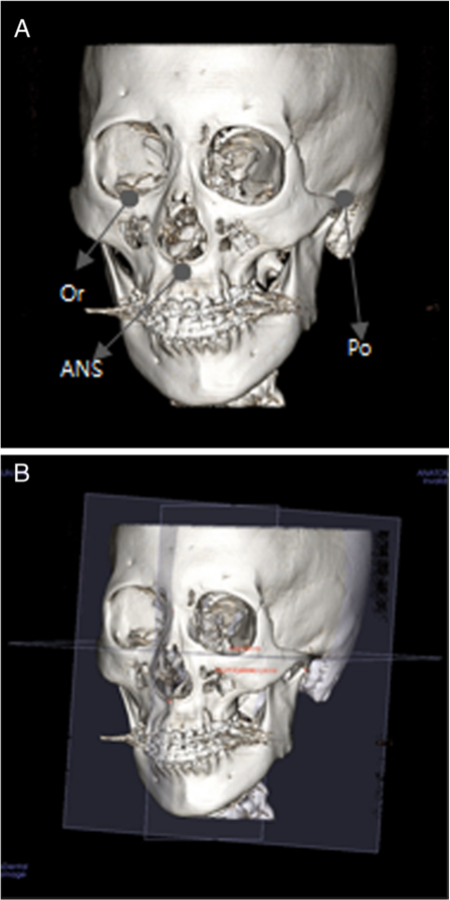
Anatomical landmarks and reference planes in 3D-CT: a reference points and b reference planes
